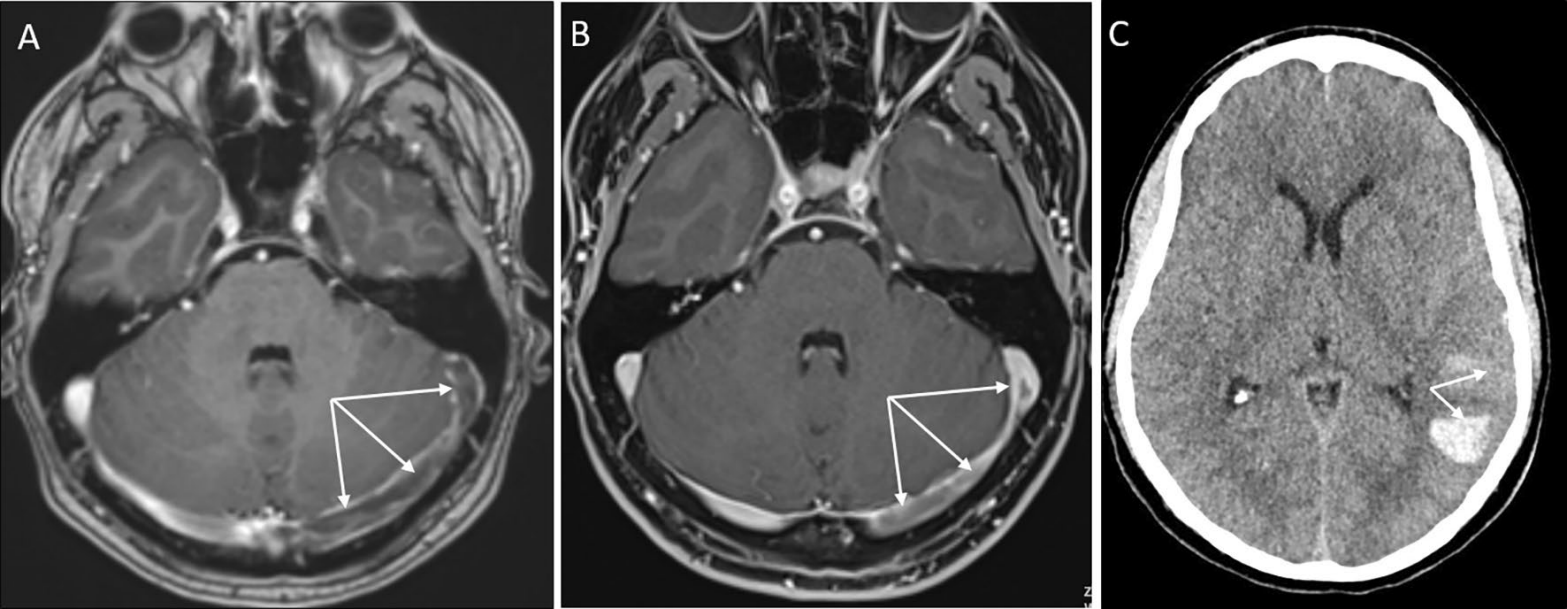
Imaging (MRI, CT) of the case report. a, b T1-weighted contrast-enhanced axial MR images on admission (a) and at 2-week follow-up (b). On admission, pronounced thrombosis is disclosed within the left transverse and sigmoid sinus, with progressive recanalization on follow-up MRI (arrows). c Plain head CT, displaying left-sided temporal hemorrhage
